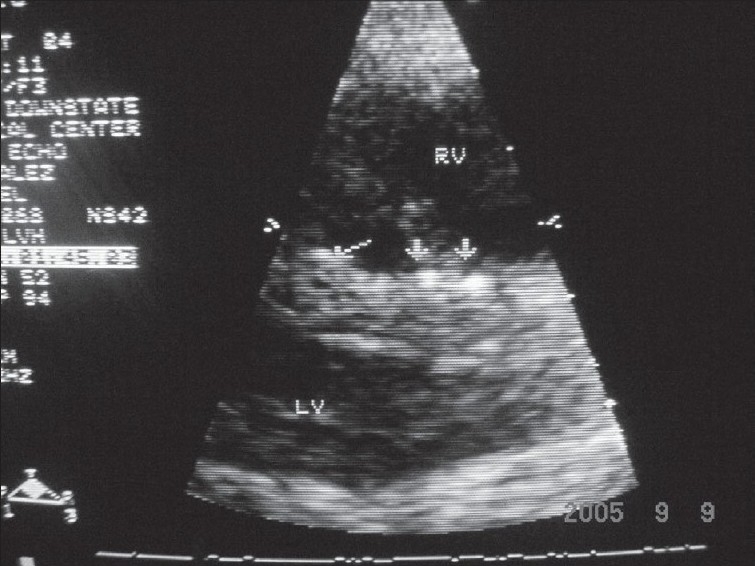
Parasternal long-axis view showing discrete small areas of calcification in interventricular septum
